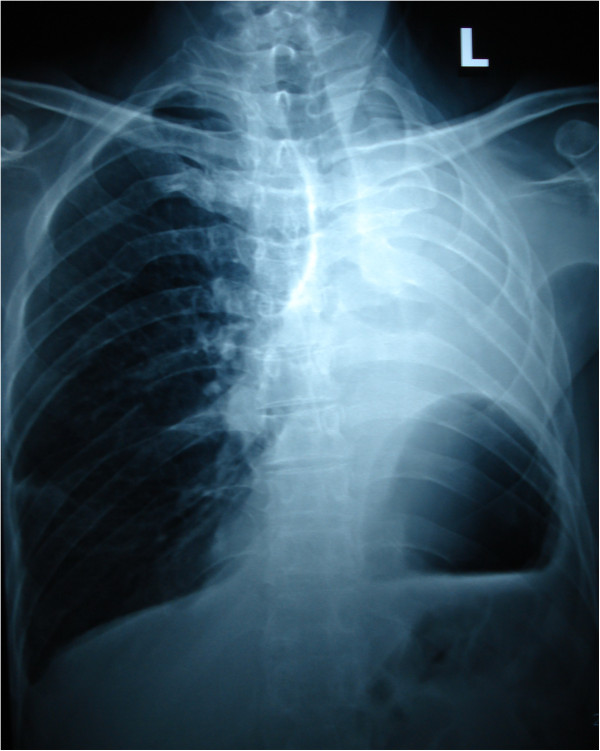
Standard radiograph done 25 days after surgery showing good expansion of the right upper and middle lobes and minimal postoperative inflammatory changes.
